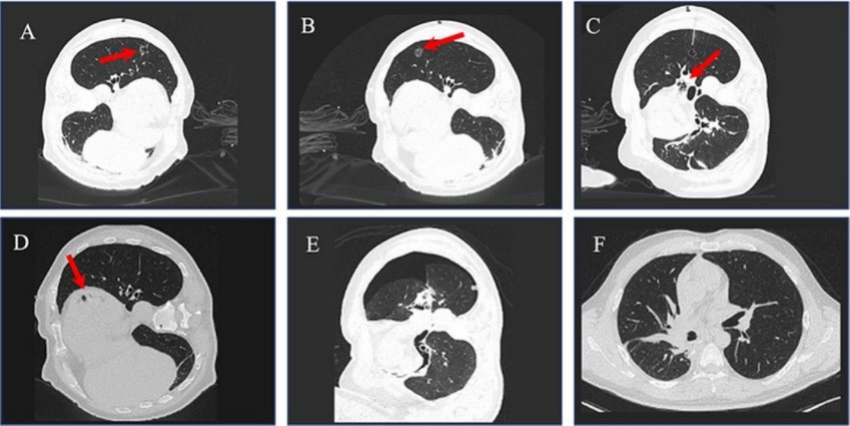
CT image of case 2. (A) Chest CT showed air-containing cavity in the posterior apical segment of left superior lung (red arrow), about 10 × 8 mm. (B) Contrast-enhanced chest CT scan a partially solid nodules in the left upper lobe (red arrow), about 21 × 16 mm. (C) Left pulmonary vein gas embolism (red arrow) at the time of puncture. (D) Left atrial gas embolism (red arrow); (E) Chest CT scan left pneumothorax after surgery. (F) Chest CT reexamination scan complete resolution of pneumothorax (no residual air/fluid) after POD 7.
